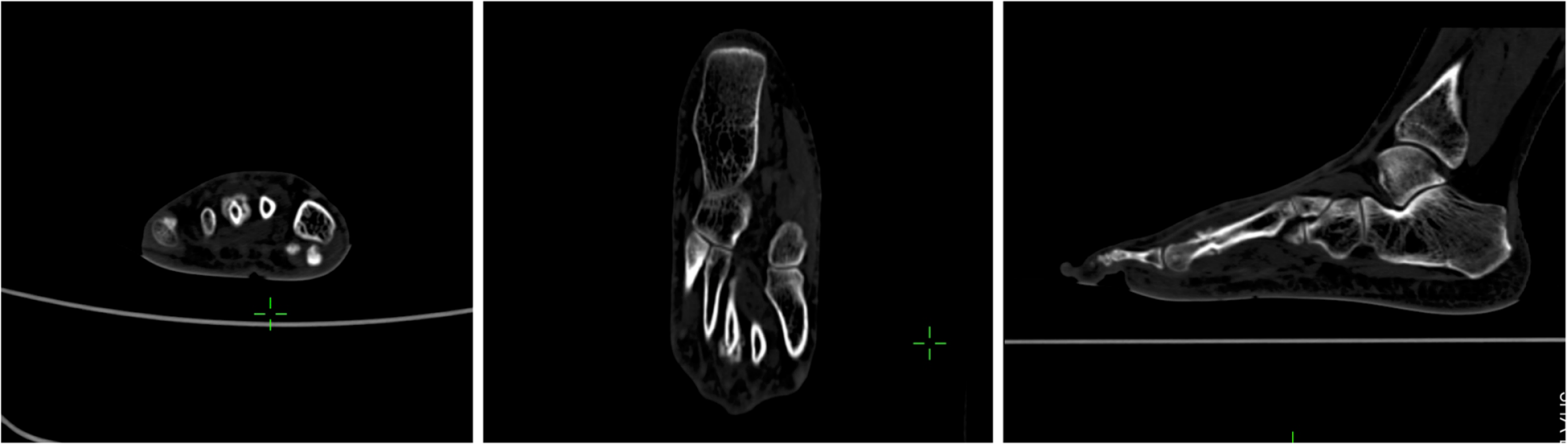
CT imaging of the right foot showing the continuity of the lesion with the cortex and interruption of bone continuity
